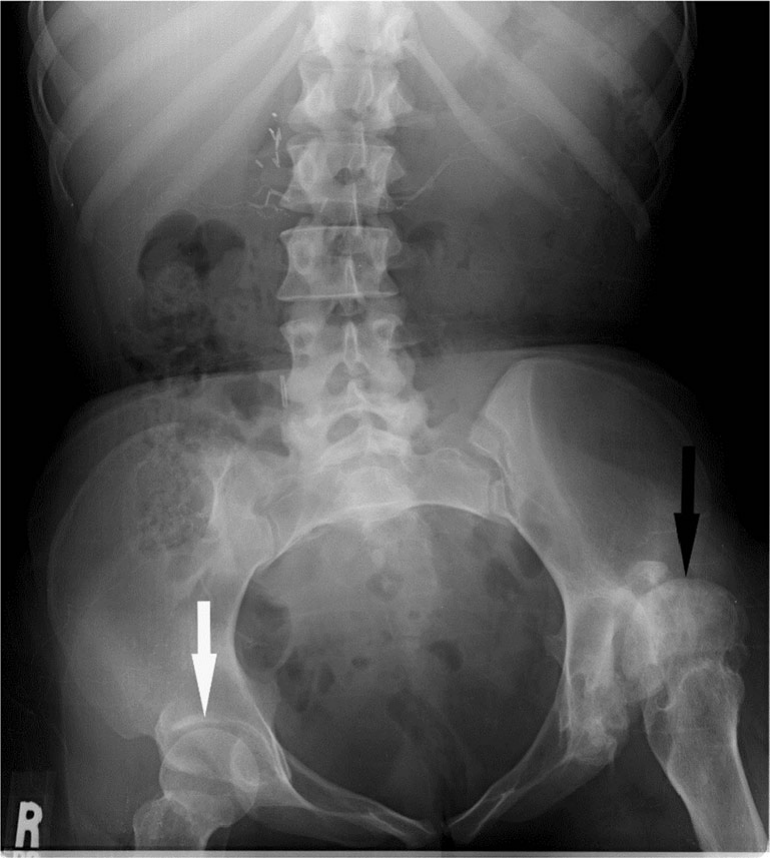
Abdominal radiograph of a 33-year-old female with thoracic SCI from a motor vehicle collision 15 years prior shows superior subluxation of the left femoral head (*black arrow*) due to spasticity. The right femur (*white arrow*) is normally aligned
